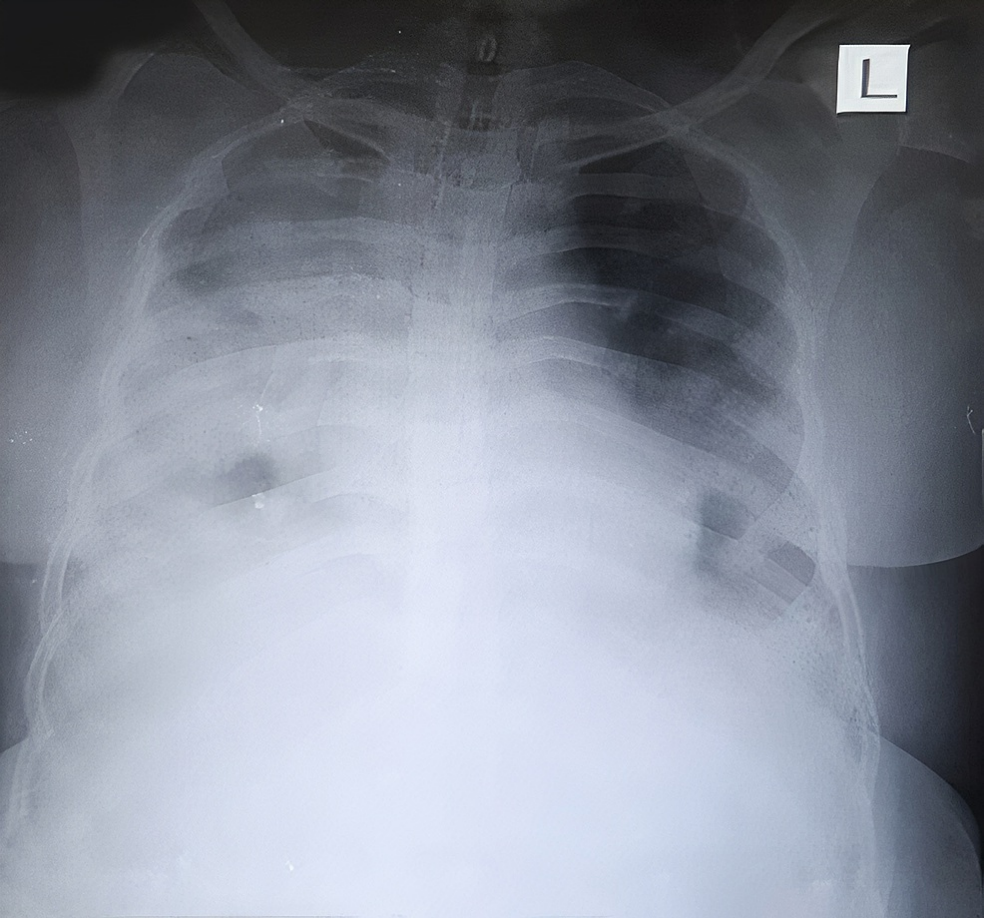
X-ray of case 1 before bevacizumab
